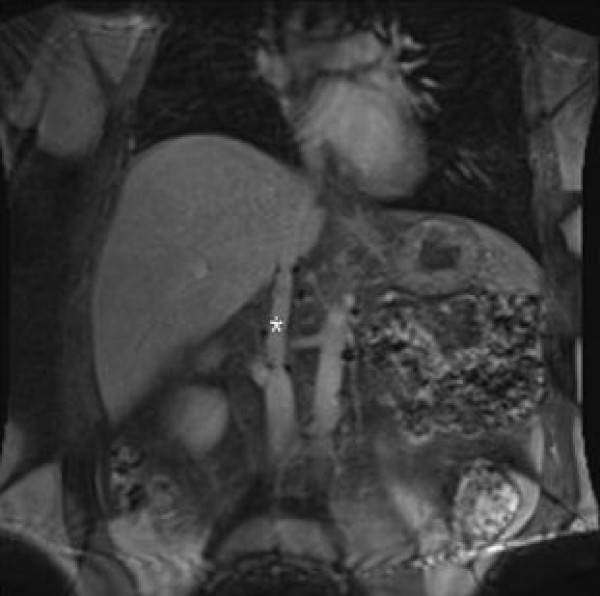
One-year follow-up magnetic resonance image shows patent polytetrafluoroethylene graft (asterisk) and no local tumor recurrence.
